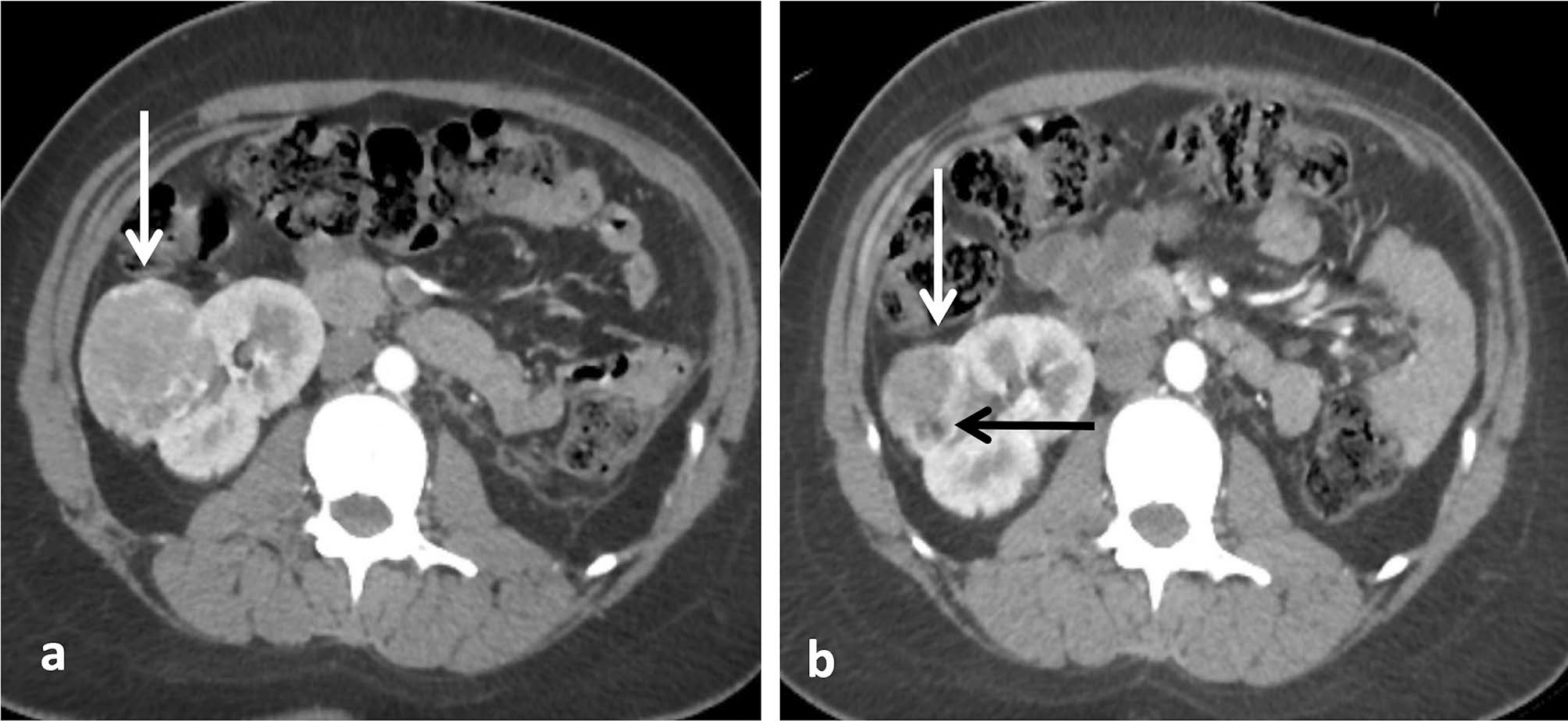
Change in AML volume and fat-containing pattern in response to sirolimus. (a) Pre-treatment axial corticomedullary-phase CT scan shows a right renal fat-poor AML (white arrow). (b) Post-treatment axial corticomedullary-phase CT image reveals tumor volume reduction (white arrow) while the fat component becomes visible (black arrow). Note that the AML total volume reduction (from 76.4 cm^3^ to 26.9 cm^3^) occurred due to diminishment of the fat-poor (from 60.1 cm^3^ to 13.0 cm^3^) and highly-vascularized (from 15.4 cm^3^ to 11.3 cm^3^) compartments.
